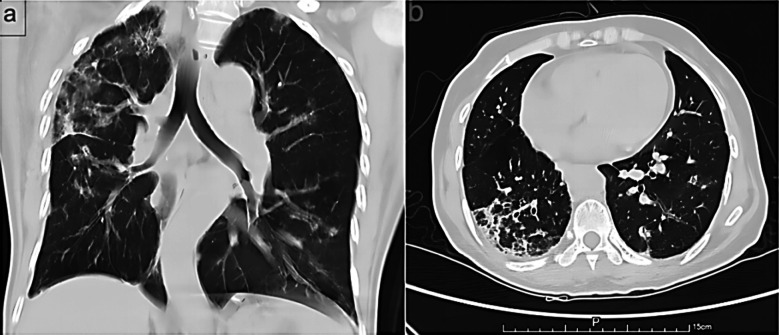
CT of the chest showed her bilateral pulmonary inflammatory infiltration, noted in the right (a, b).
